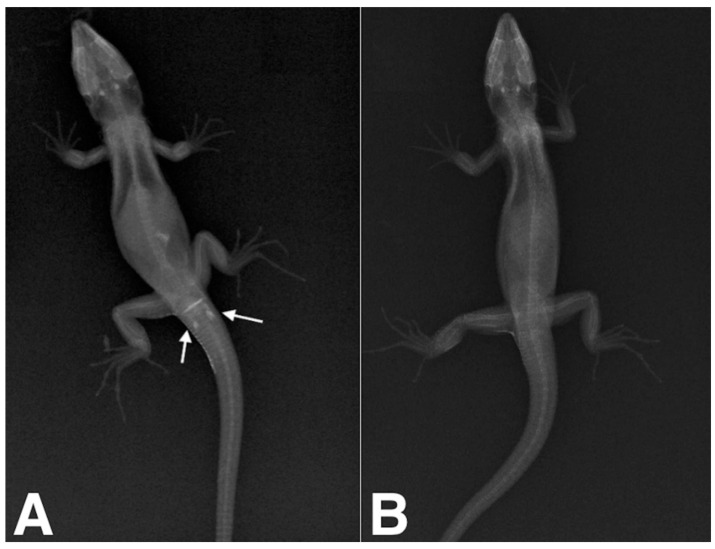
(A) Positive contrast radiographic study of a four-month old, 8 cm SVL Sierra Nevada lizard (*Timon nevadensis*); dorsoventral projection. White arrows indicate both the right and left hemipenes. (B) Negative contrast radiographic study of a four-month old Sierra Nevada lizard (*Timon nevadensis*); dorsoventral projection. There is no evidence of hemipenes in the postcloacal region.
